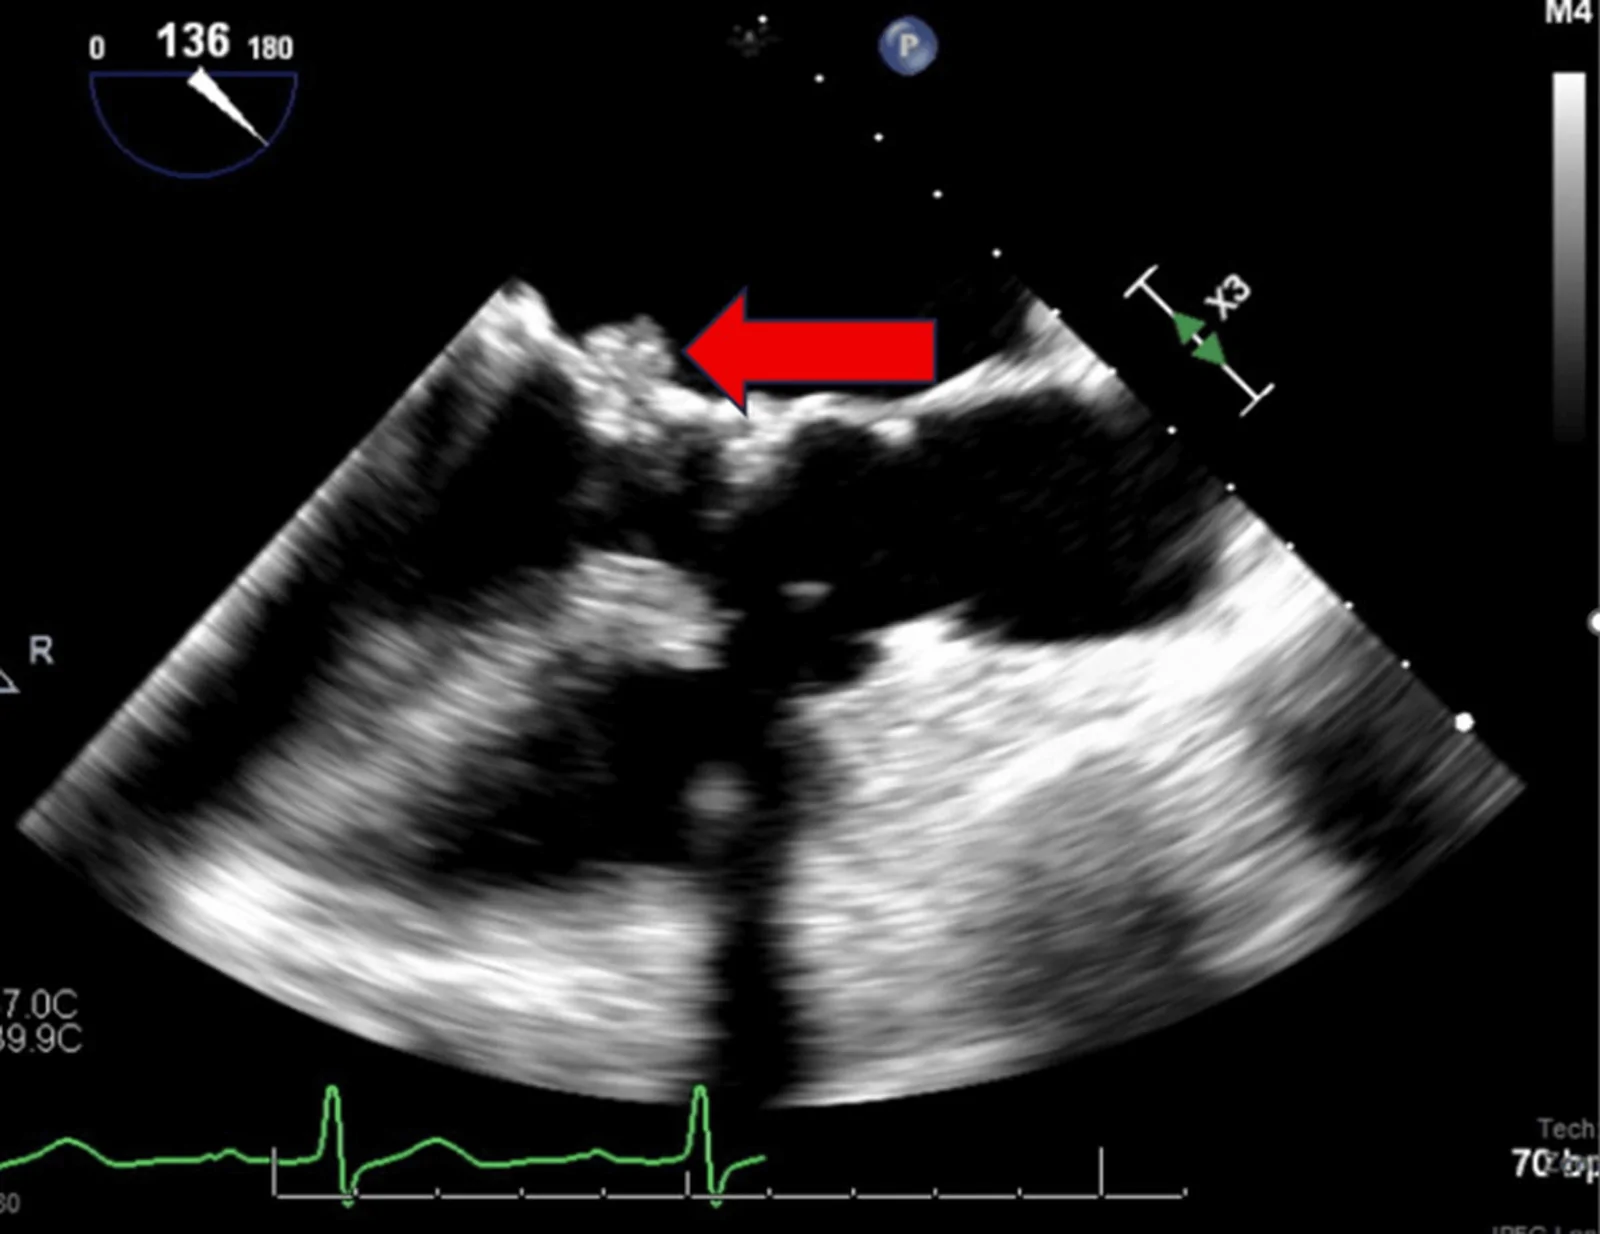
Initial transesophageal echocardiogram (TEE), mid-esophageal four-chamber view, demonstrating irregular thickening of a bioprosthetic mitral valve with a mobile, filamentous density (~10 mm), concerning for vegetation (red arrow)
